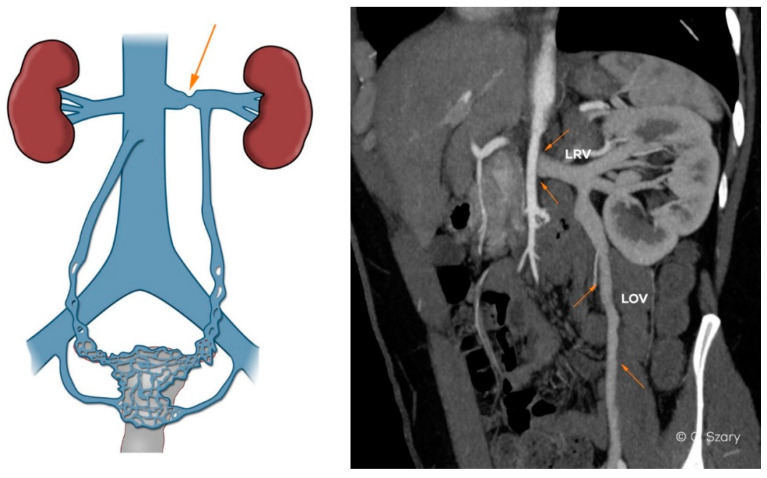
Typical anatomy of nutcracker syndrome. Insufficiency of LOV on computed tomographic venography (CTV) image.
